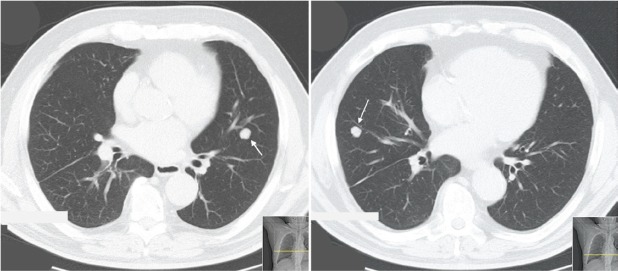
Thoracic CT scan (patient #3) exhibiting bilateral nodes. Arrow indicates lesion in the left lung (apical segment of the lower lobe) and right lung (anterior segment of the lower lobe).
